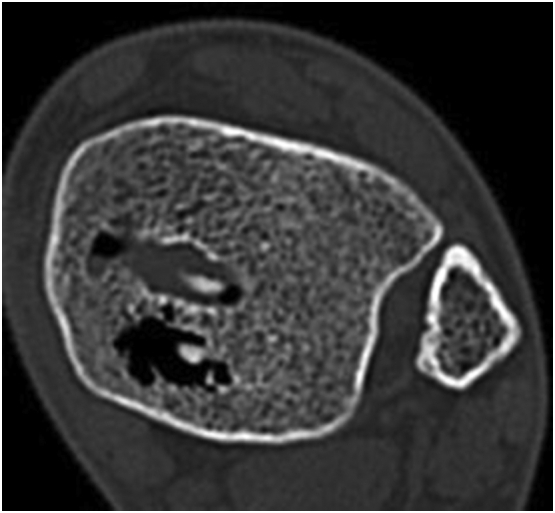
52 weeks timepoint CT scan of the ankle; new bone formation at the radiolucency around the screw, significant degradation of the implant.
